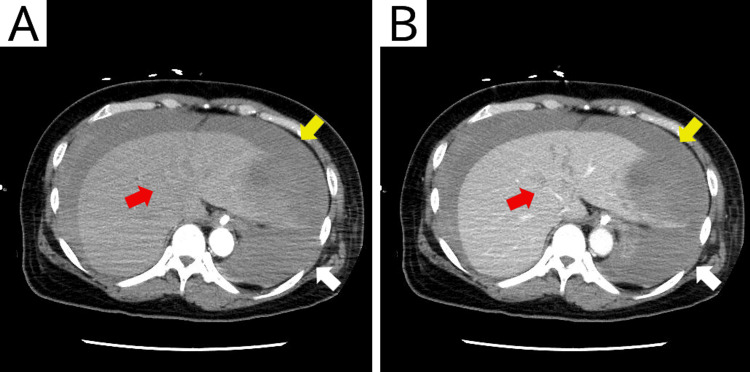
Contrast-enhanced computed tomography of the abdomen (A: arterial phase; B: portal venous phase) shows hepatic lacerations (red arrow) with associated hemoperitoneum (white arrow) and hepatic hematoma, including subcapsular and intraparenchymal components (yellow arrow). The images reveal no contrast extravasation.
